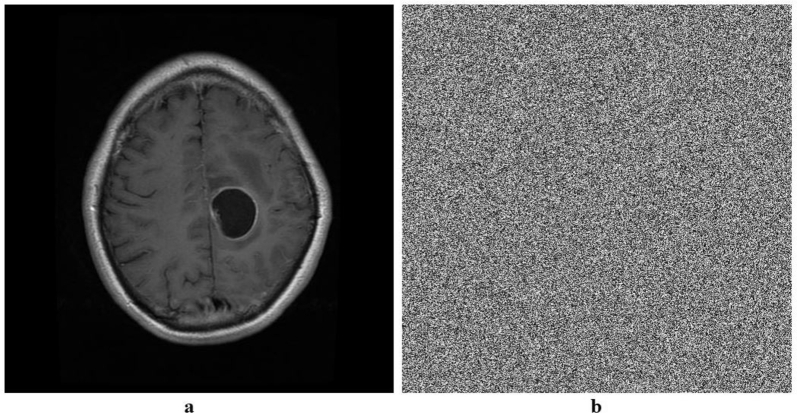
Plain and encrypted MRI image.
